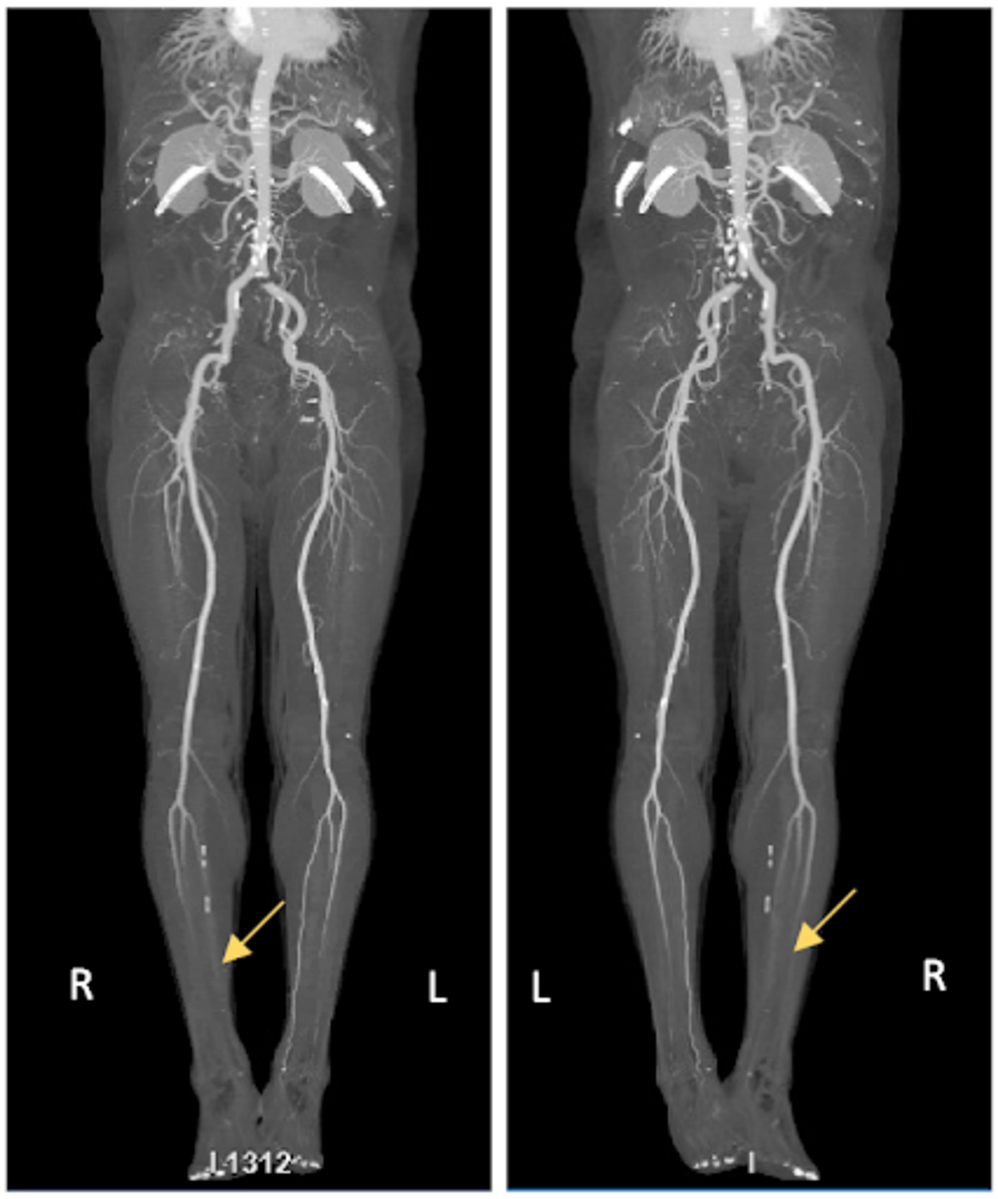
Anterior and posterior images from the CTA with iliofemoral run-off showing decreased patency of the vessels of the lower extremities, right worse than left. CTA, computed tomography angiogram
